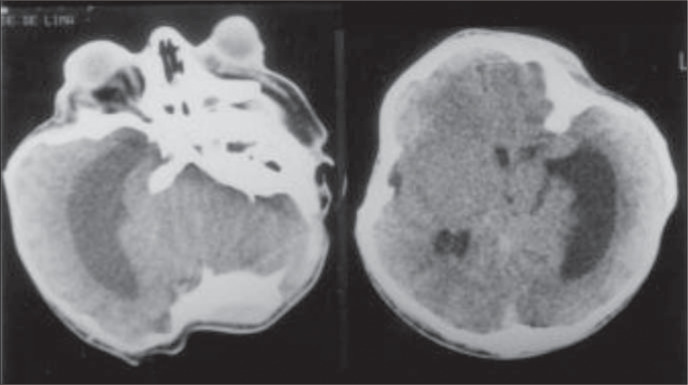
Computed tomography findings - dilatation of the lateral ventricles, cranial deformities and shallow orbit.
